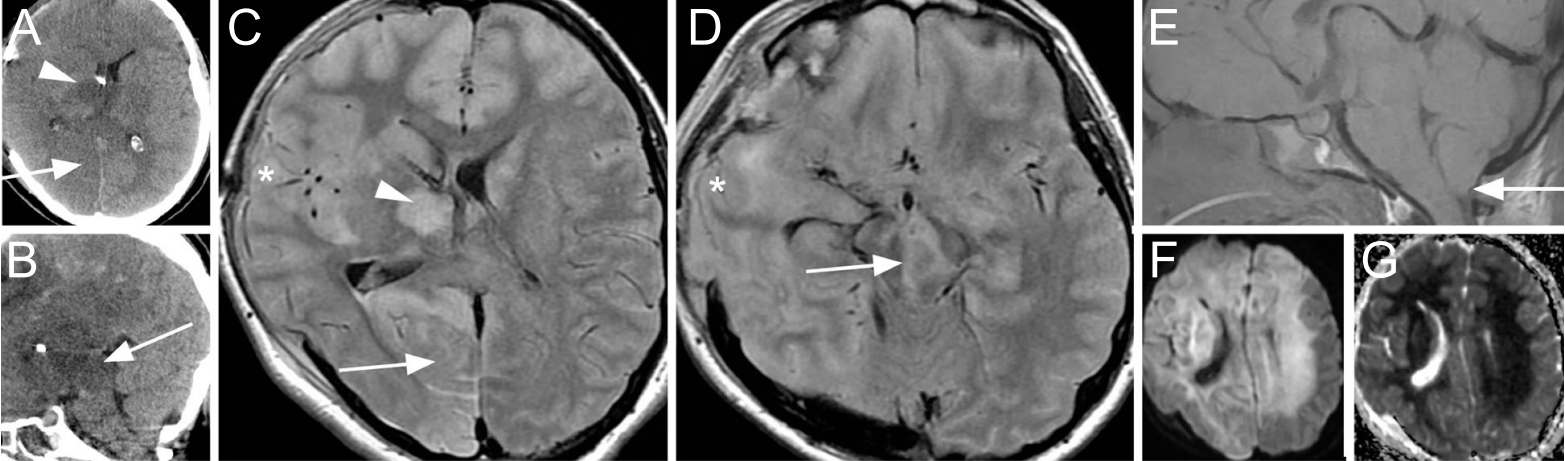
a, b Noncontrast head CT axial (a) and sagittal (b) images two days postoperatively showing hypodensities in a ‘top of the basilar’ injury distribution, affecting the thalami (A, arrowhead), right PCA territory (A, arrow) and midbrain (B, arrow). c, d Axial FLAIR MRI sequences ten days postoperatively showing evolution of the ischemic injuries to the thalami (C, arrowhead), right PCA territory (C, arrow), and midbrain (D, arrow). Herniation of the brain through the craniectomy defect (C and D, asterisks) and downward through the foramen magnum (E, arrow) suggests intracranial hypertension. f, g Axial diffusion-weighted sequence (f) and corresponding apparent diffusion coefficient map (g) show diffuse acute supratentorial deep white matter cytotoxic edema
